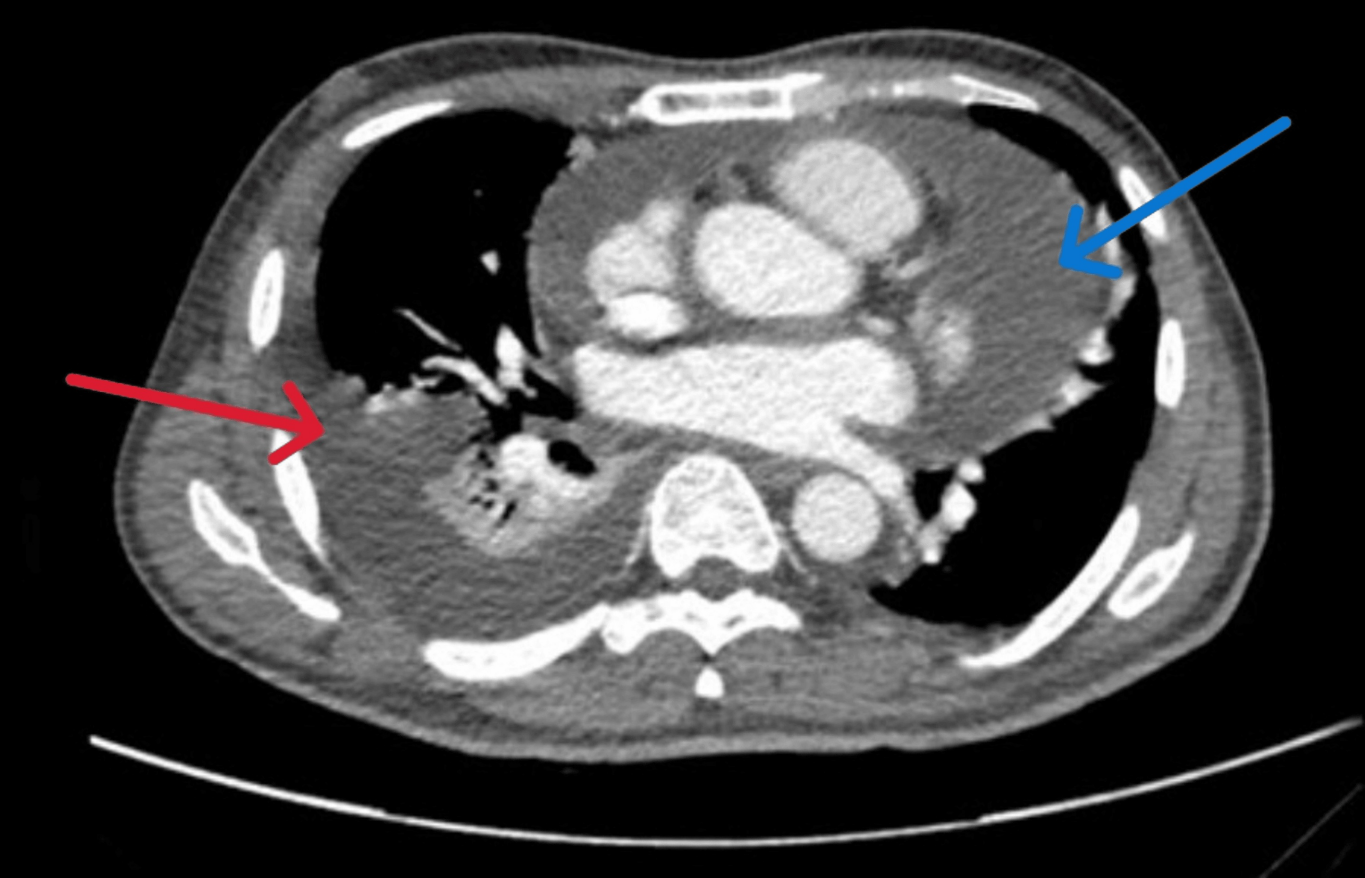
CT chest (axial view) showing right-sided pleural effusion (red arrow) and pericardial effusion (blue arrow).
